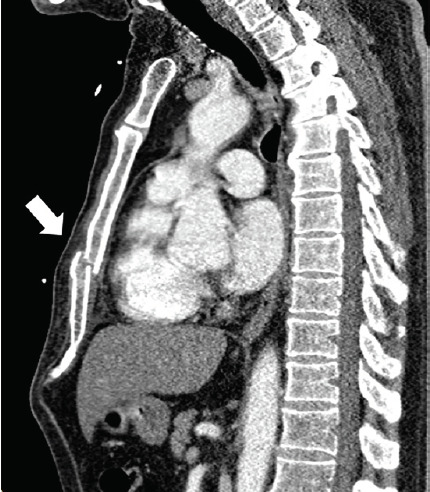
Chest computed tomography showing a displaced sternal fracture (arrow).
